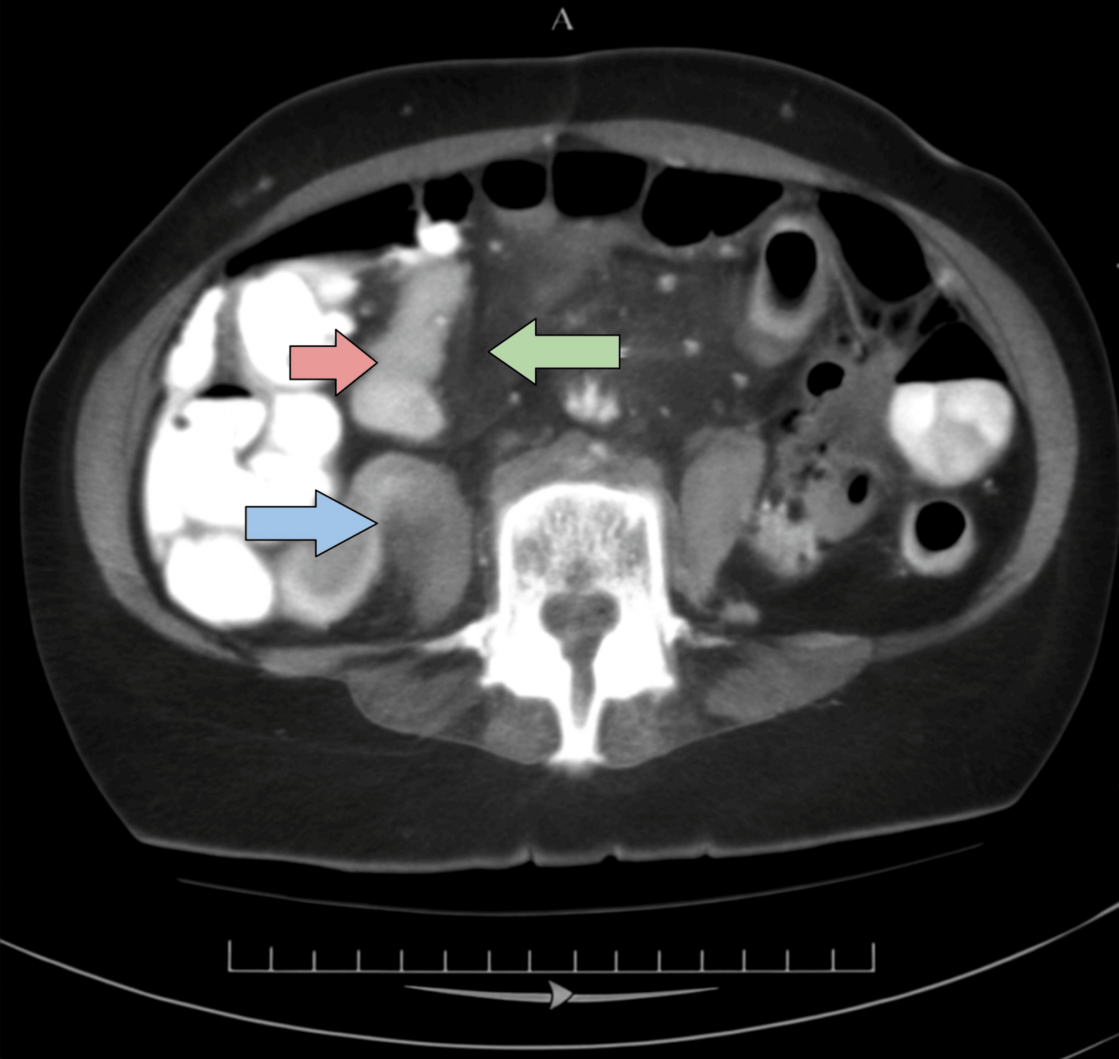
Axial contrast-enhanced CT scan of the abdomen. Axial contrast-enhanced computed tomography of the abdomen demonstrating mechanical small bowel obstruction. The red arrow indicates air-fluid levels within a proximal small bowel loop. The blue arrow highlights a dilated small bowel segment, while the green arrow marks the transition point between the dilated proximal and collapsed distal bowel in the mid-abdomen. No radiologic signs of ischemia or perforation are evident.
